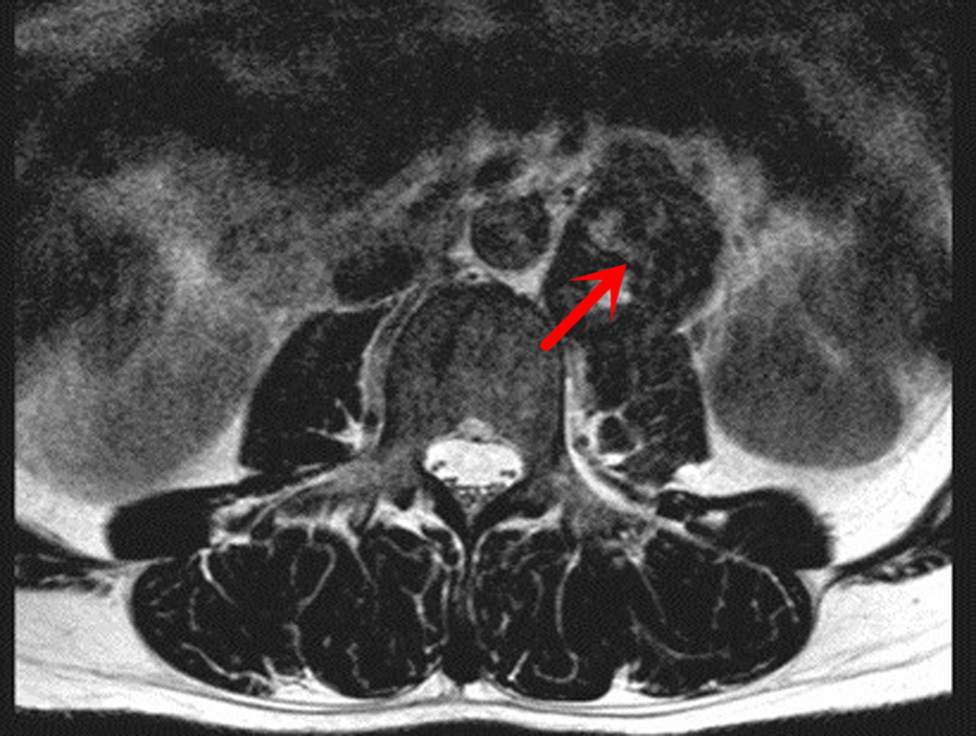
MRI T2WI cross section: quasi-circular abnormal signal area in the left front of the second and third lumbar vertebrae. The T2WI signals were mixed and had uneven enhancement.
